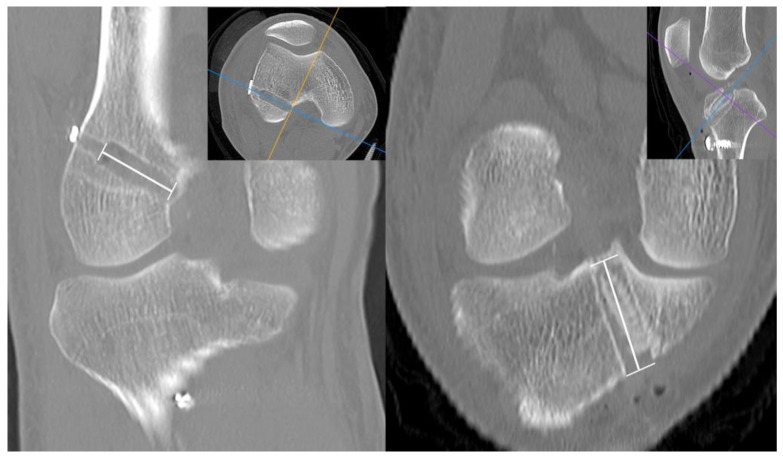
Measurement of the length of femoral and tibial tunnel on CT scan.
